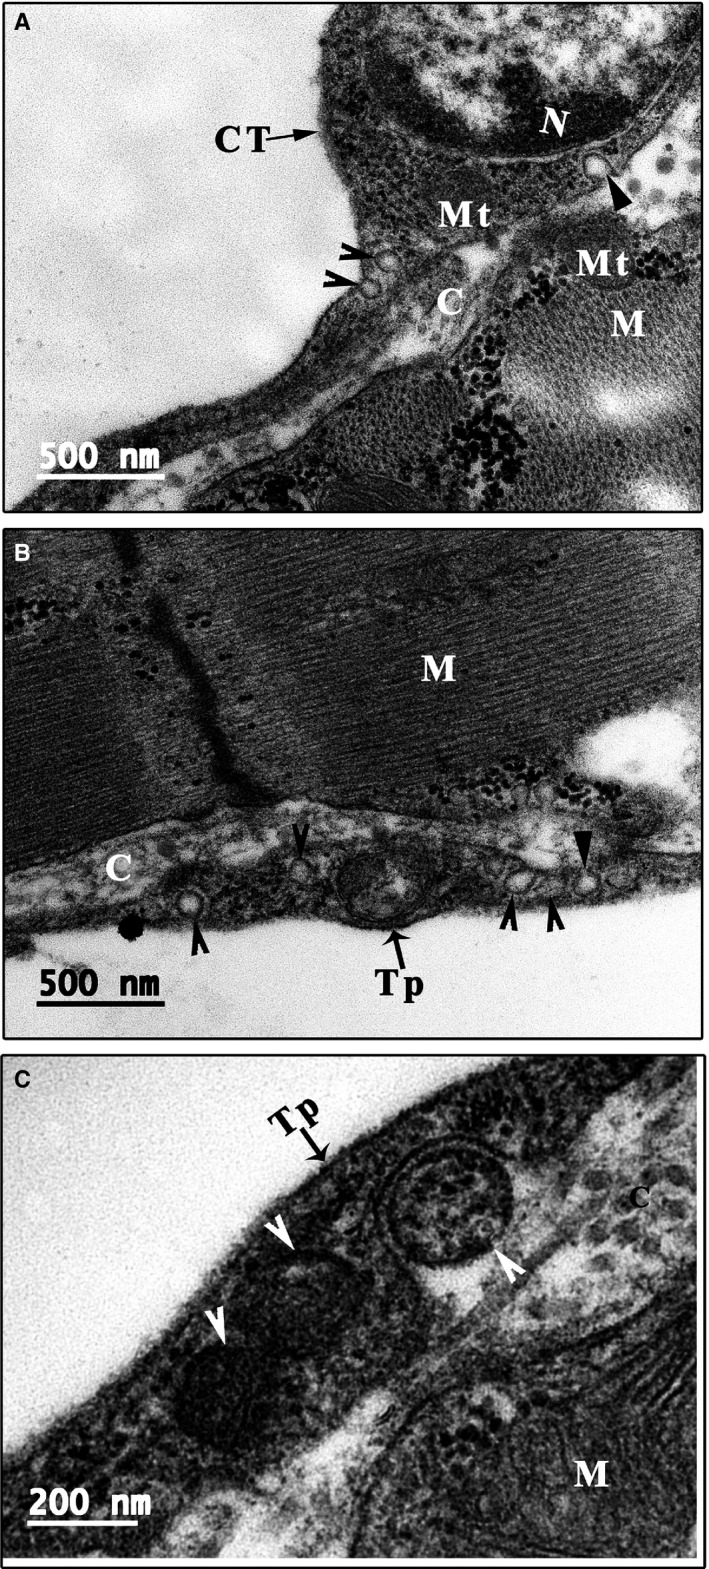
Vesicles and caveolae of CTs. Many vesicles (arrowhead) or coated vesicles (white arrowhead) are present in the telopodes. In addition, many caveolae are present in the membrane of the cell body and telopode (small triangle). The opening site of caveolae faces the extracellular space, and some vesicles or coated vesicles are located near the opening site of caveolae (A‐C). C: Collagen and extracellular matrix; CT: Cardiac telocyte; M: Cardiomyocyte; Mt: Mitochondria; N: Nucleus. Scale bar: Size as shown in the figures; Tp: Telopode
